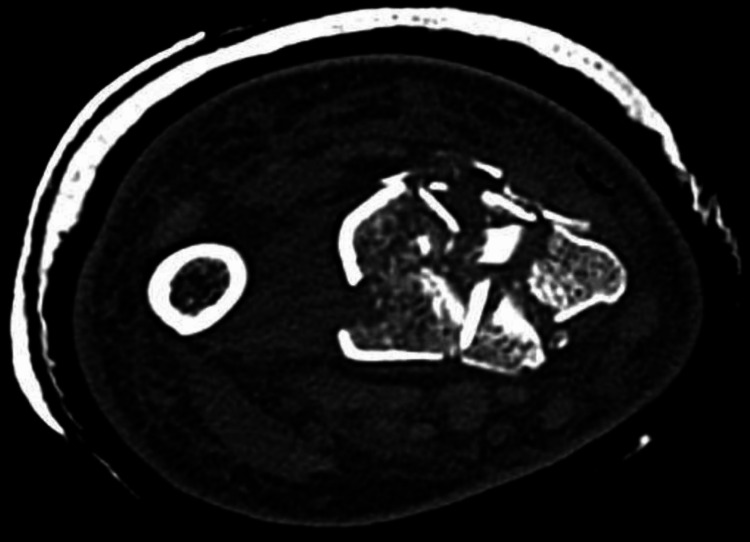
Axial computed tomography (CT) scan image This demonstrates the significant comminution of the distal radius and displacement of the lunate and scaphoid fossae.
